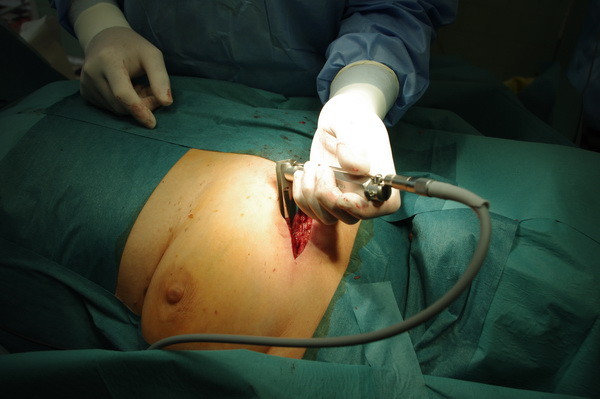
The position of patient and the place of incision of left side mediastinoscope-controlled parasternal pericardial fenestration.
